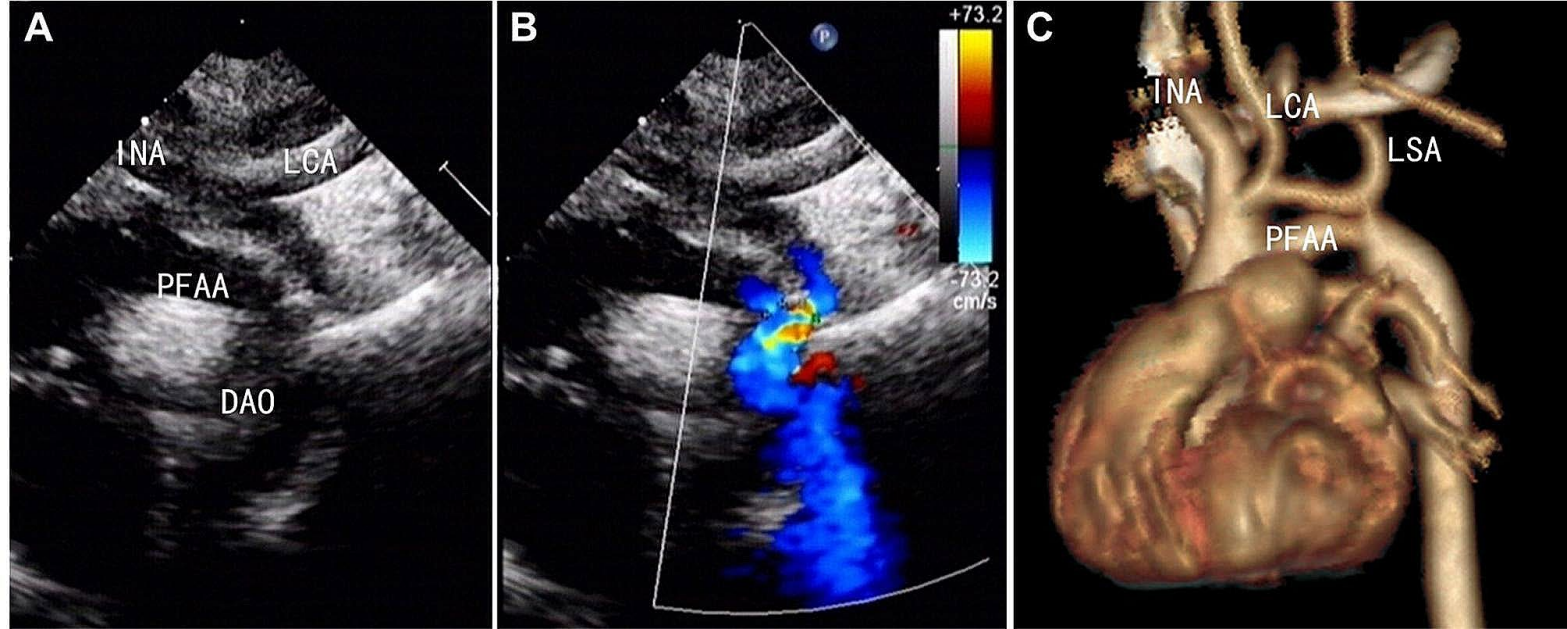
Case 1, Weinberg type A PFAA combined with aortic coarctation. (A & B): Echocardiography showing the fifth aortic arch originating from the distal ascending aorta and two coexisting and paralleled aortic arches connecting to the descending aorta. (C): Confirmation via computed tomography. DAO: descending aorta; INA, innominate artery; LCA, left common carotid artery; PFAA: persistent fifth aortic arch
